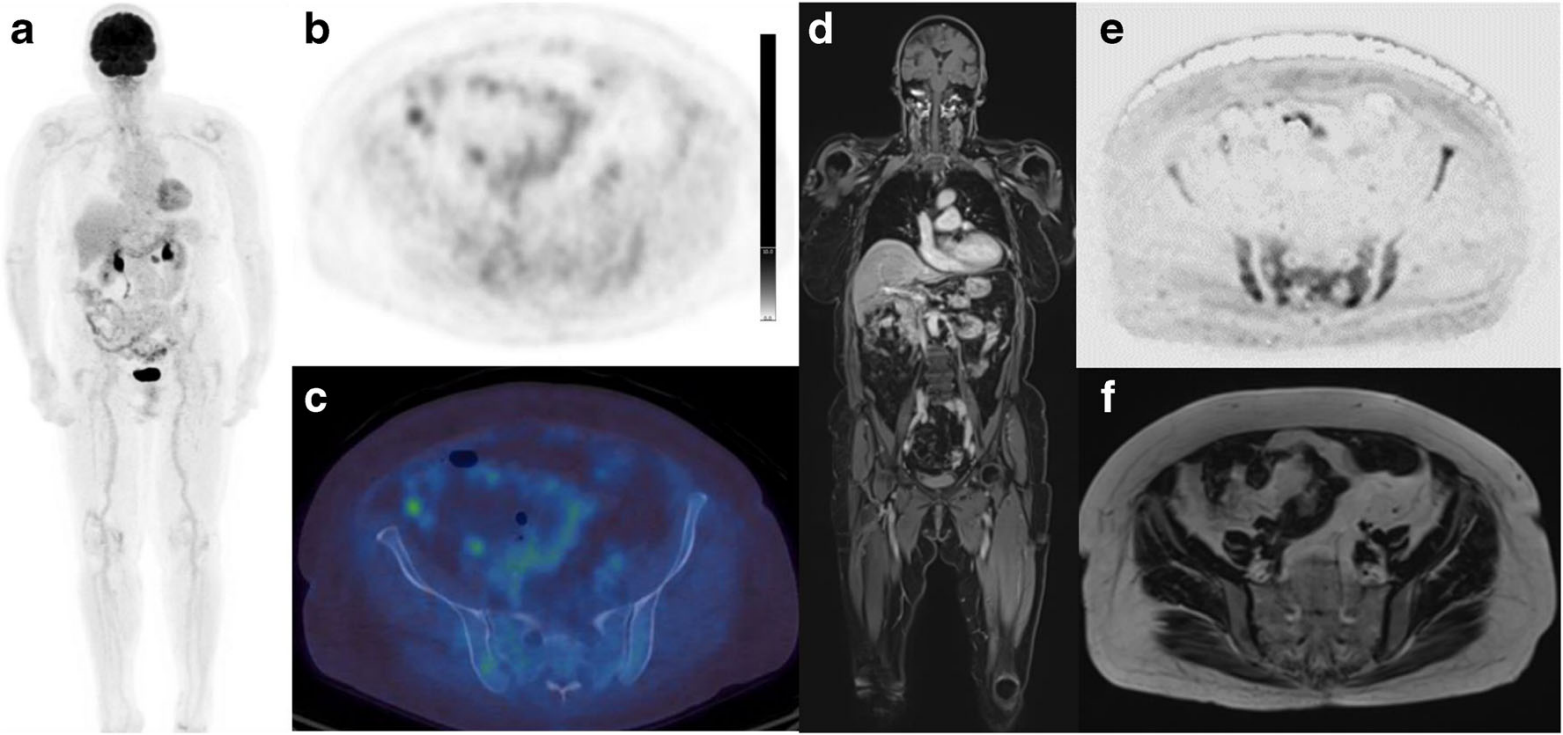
Eighty-three-year-old female with smouldering myeloma, rising serum paraprotein and 15% bone marrow infiltration. 18F-FDG PET/CT (a: Whole body MIP; b: Axial PET image; c: Axial fused image) and WBMRI (d: Coronal contrast enhanced T1-weighted fat-suppressed Dixon image; e: Axial inverted diffusion b900 image; f: Axial T2-weighted image) are shown. Pelvic focal lesions and marrow infiltration seen on the diffusion and contrast enhanced T1-weighted images are not as easily detected on 18F-FDG PET/CT (bone marrow: liver FDG uptake ratio <1.0)
